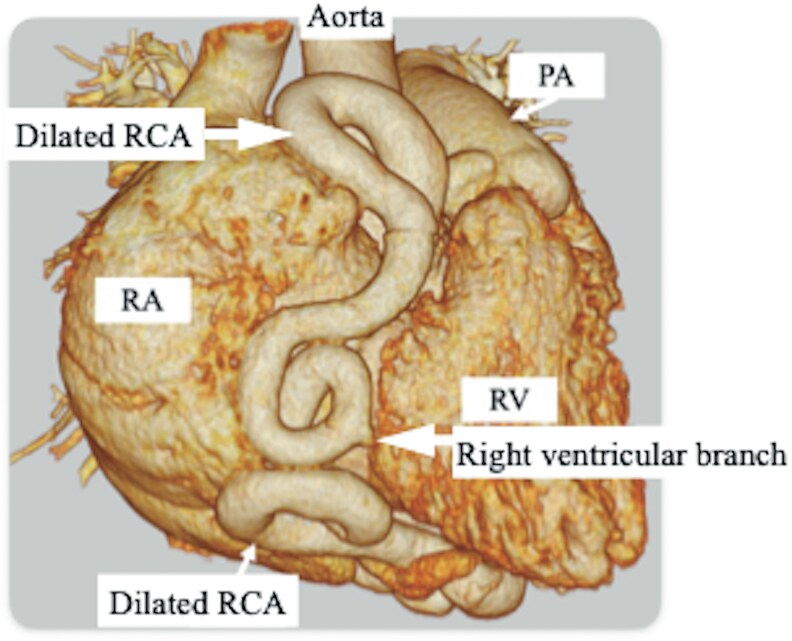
Preoperative computed tomography revealing an aneurysm in the right coronary artery. A small fistula arose from the left circumflex artery as a side branch. Both fistulas drained into the coronary sinus.
